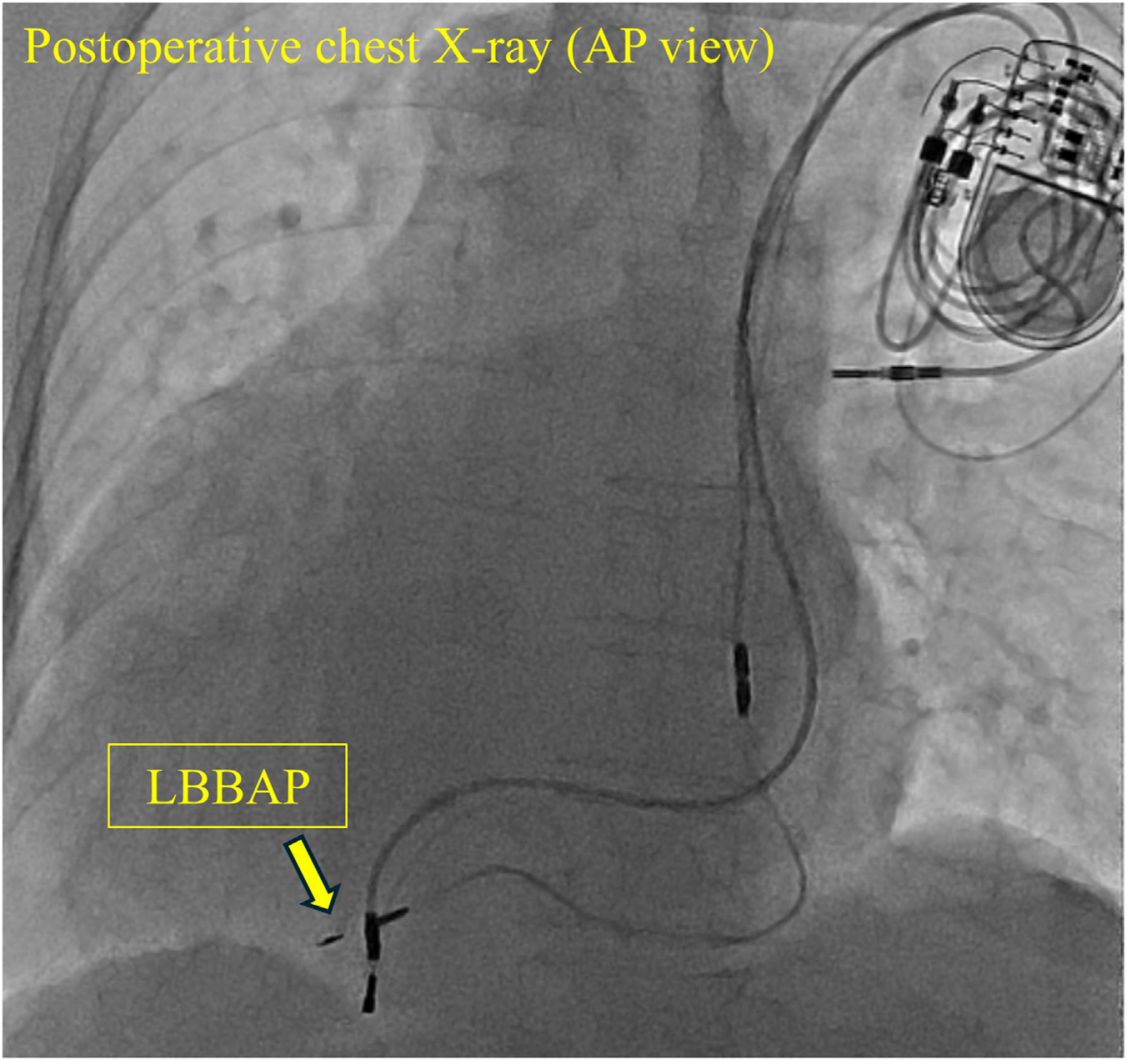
Chest radiograph after LBBAP. RV lead (*yellow arrow*) at upper site of previous one. AP = anteroposterior; LBBAP = left bundle branch area pacing; RV = right ventricle.
